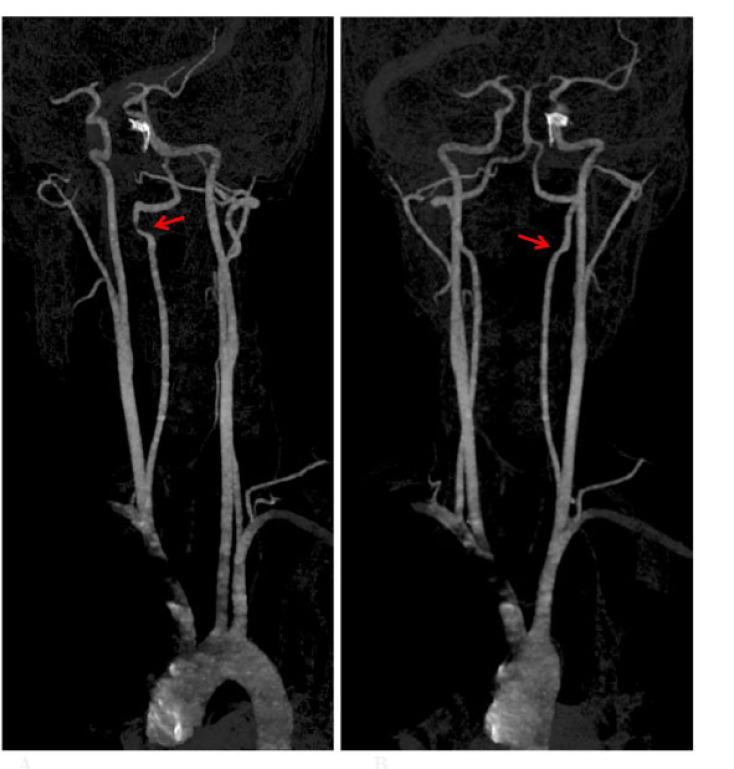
Cervical CTA. (A) Moderate stenosis of the right VA at the V3 segment. (B) Mild stenosis of the left VA at the V3 segment.
